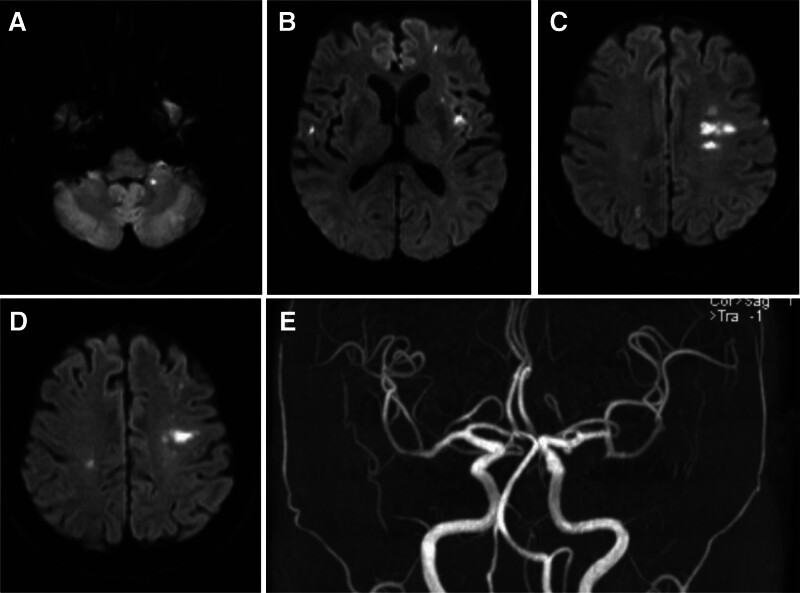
Images of Case 1. (A) MRI shows acute cerebral infarctions in the left cerebellar hemisphere. (B) MRI shows acute cerebral infarctions in the left frontotemporal lobe, basal ganglia region, and right temporal lobe. (C) MRI shows acute cerebral infarctions in the left frontoparietal lobe and right parieto-occipital lobe. (D) MRI shows acute cerebral infarctions in the bilateral parietal lobes. (E) There were no obvious abnormalities on time-of-flight MRA scanning. MRA = magnetic resonance angiography, MRI = magnetic resonance imaging.
